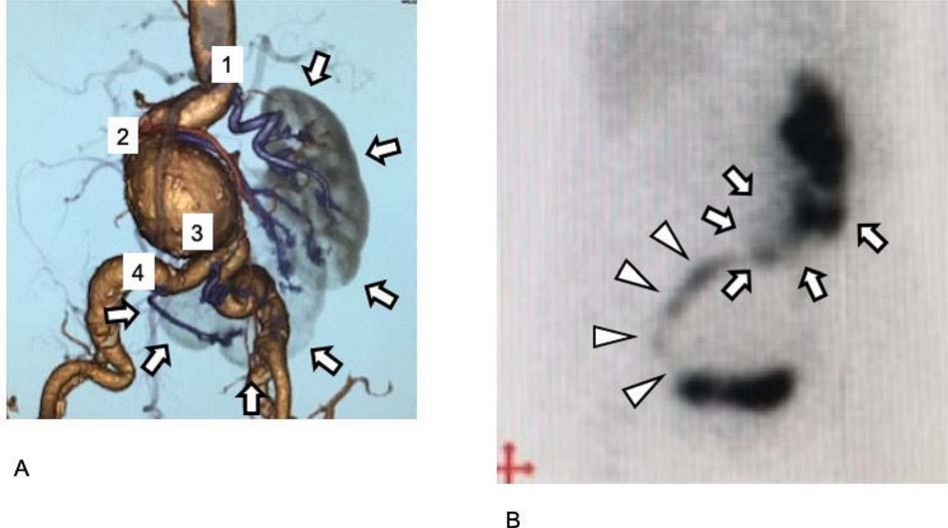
Preoperative images of the aneurysm and the kidney. (A) Preoperative three-dimensional enhanced CT image. Four RA are visible (purple and numbered). Cross-fused renal ectopia was diagnosed, and the right kidney had migrated to the left side. The kidney is L-shaped and indicated in gray (arrow). (B) Preoperative 99mTc-MAG3 renogram. The L-shaped kidney is well observed (arrow). The urinary tract arises from the left side of the kidney and progresses to the right side, and then finally runs into the bladder at the right side (arrowhead).
